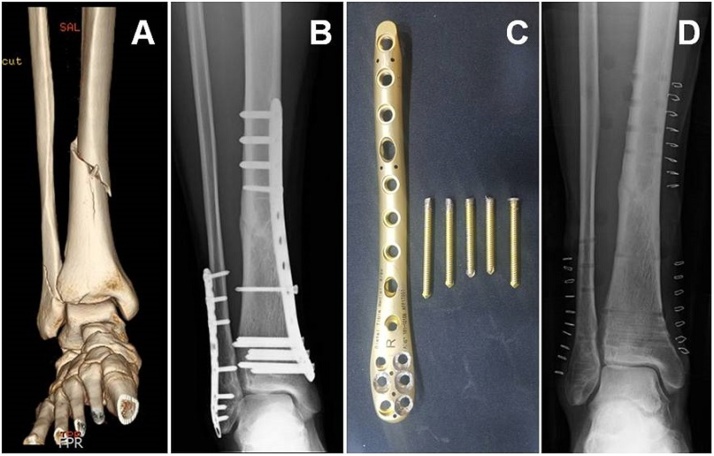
(A) 29-year-old male sustained fractures of the right tibial shaft and lateral malleolus, and underwent surgical treatment using a locking plate at another hospital. (B) Then, he was transferred to our hospital, and following identification of bony union, plate removal was scheduled. (C) Intraoperatively, multiple heads of the locking screw were damaged and could not be removed. Thus, we used a screw extractor (IRWIN^®^, Huntersville, NC, USA), a non-medical instrument that had been sterilized. Through drilling, the plate was separated from the screws and could be removed first. The remaining shafts of the screws were removed with a vice grip. (D) Postoperative radiograph reveals that the plate and screws were removed without substantial complications.
